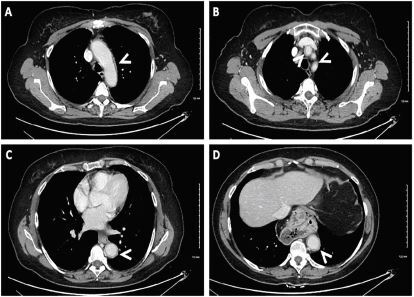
Axial views of computed tomography chest and abdomen demonstrating prominent wall thickening of the aorta (white arrows). (A) aortic arch, (B) left common carotid artery and left subclavian artery, and (C and D) descending aorta which all demonstrate diffuse and circumferential wall thickening without luminal narrowing, suggestive of phase II aortitis.
